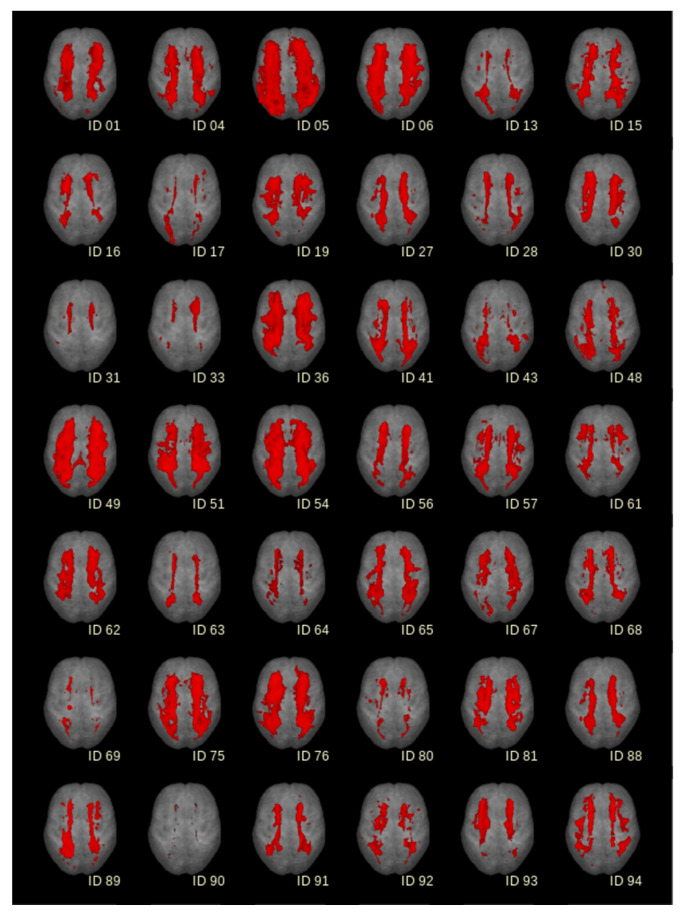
White matter hyperintensities (WMH) for 42 patients detected from FLAIR-MRI recordings in axial projection views. For comparability, individual results were normalised to the Montreal Neurological Institute (MNI) coordinate frame and displayed on the same morphological background (segmented averaged brain).
